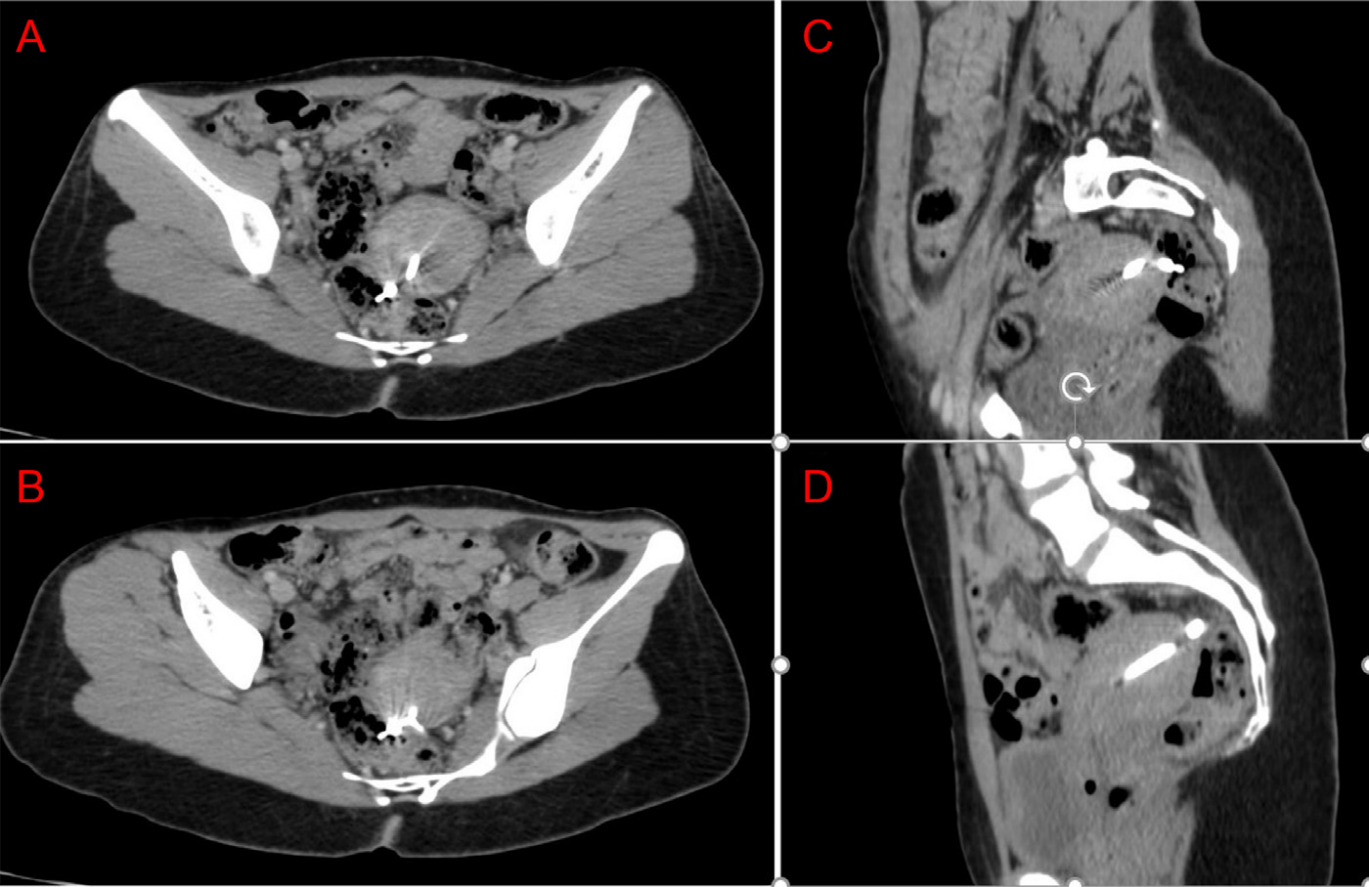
Axial (A,B) and sagittal (C,D) CT, showing an intrauterine device, which has migrated from the lumen of the uterus through the uterine wall and part of the device is now intra-rectally located.
